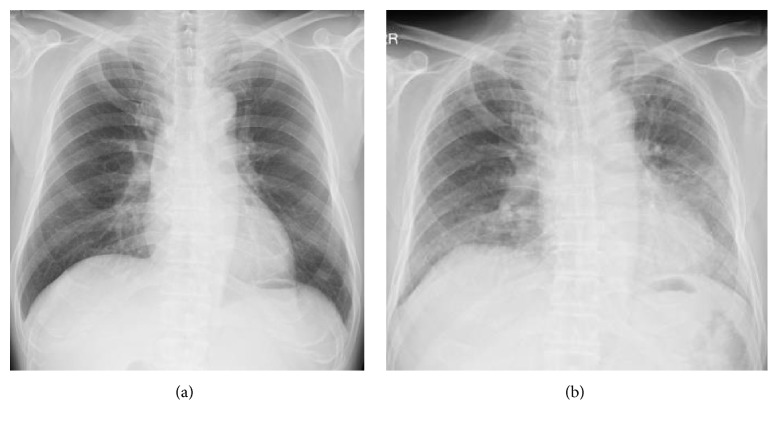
Chest X-ray images at the time of diagnosis with BD (a) and at the first time appearance of dyspnea (b).
